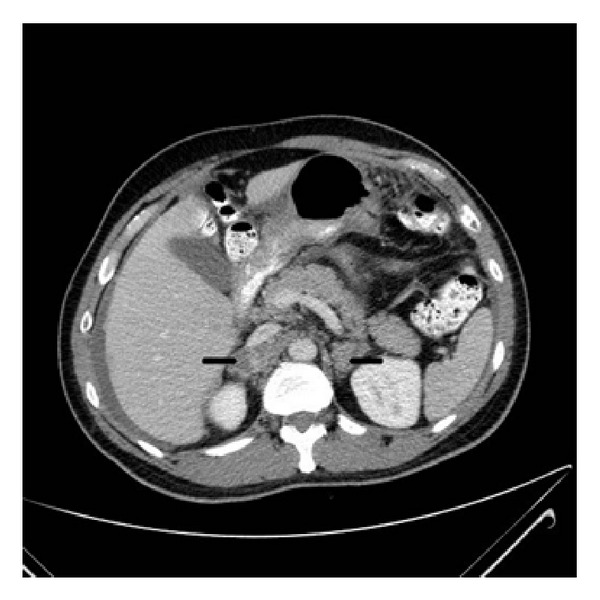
CT scan of the abdomen and pelvis with oral and intravenous contrast showing bilateral adrenal enlargement (black arrows).
